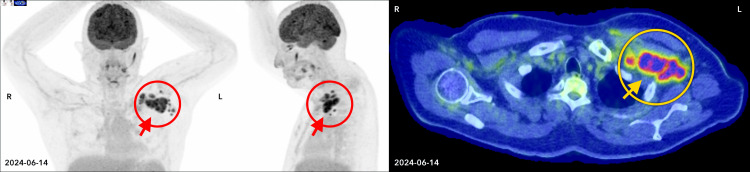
Pre-BV-AVD imaging. Pre-BV-AVD FDG positron emission tomography-computed tomography (PET-CT) maximum intensity projection (axial and coronal, left) and transverse section (right) demonstrating an intensely FDG-avid left retropectoral lymph node conglomerate (indicated by arrows). BV-AVD: brentuximab vedotin, adriamycin, vinblastine, and dacarbazine; FDG: fluorodeoxyglucose.
